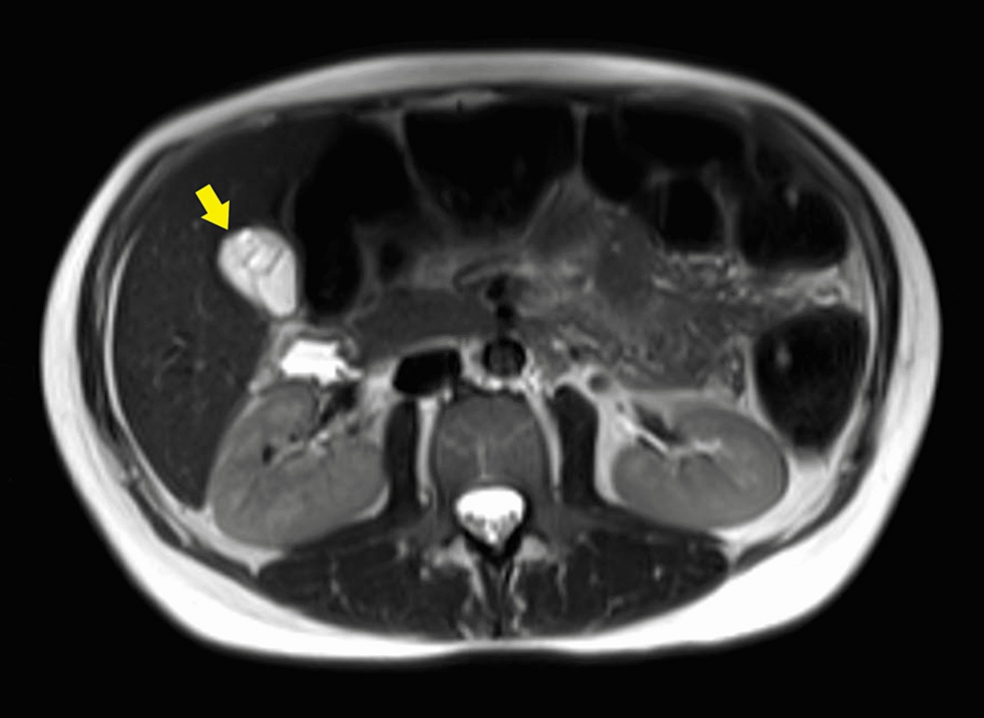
Septations seen in gallbladder on MRI abdomen/pelvis (yellow arrow). MRI abdomen/pelvis without IV contrast was obtained to rule out other abdominal pathologies and also demonstrates septations in the gallbladder. MRI: Magnetic resonance imaging
